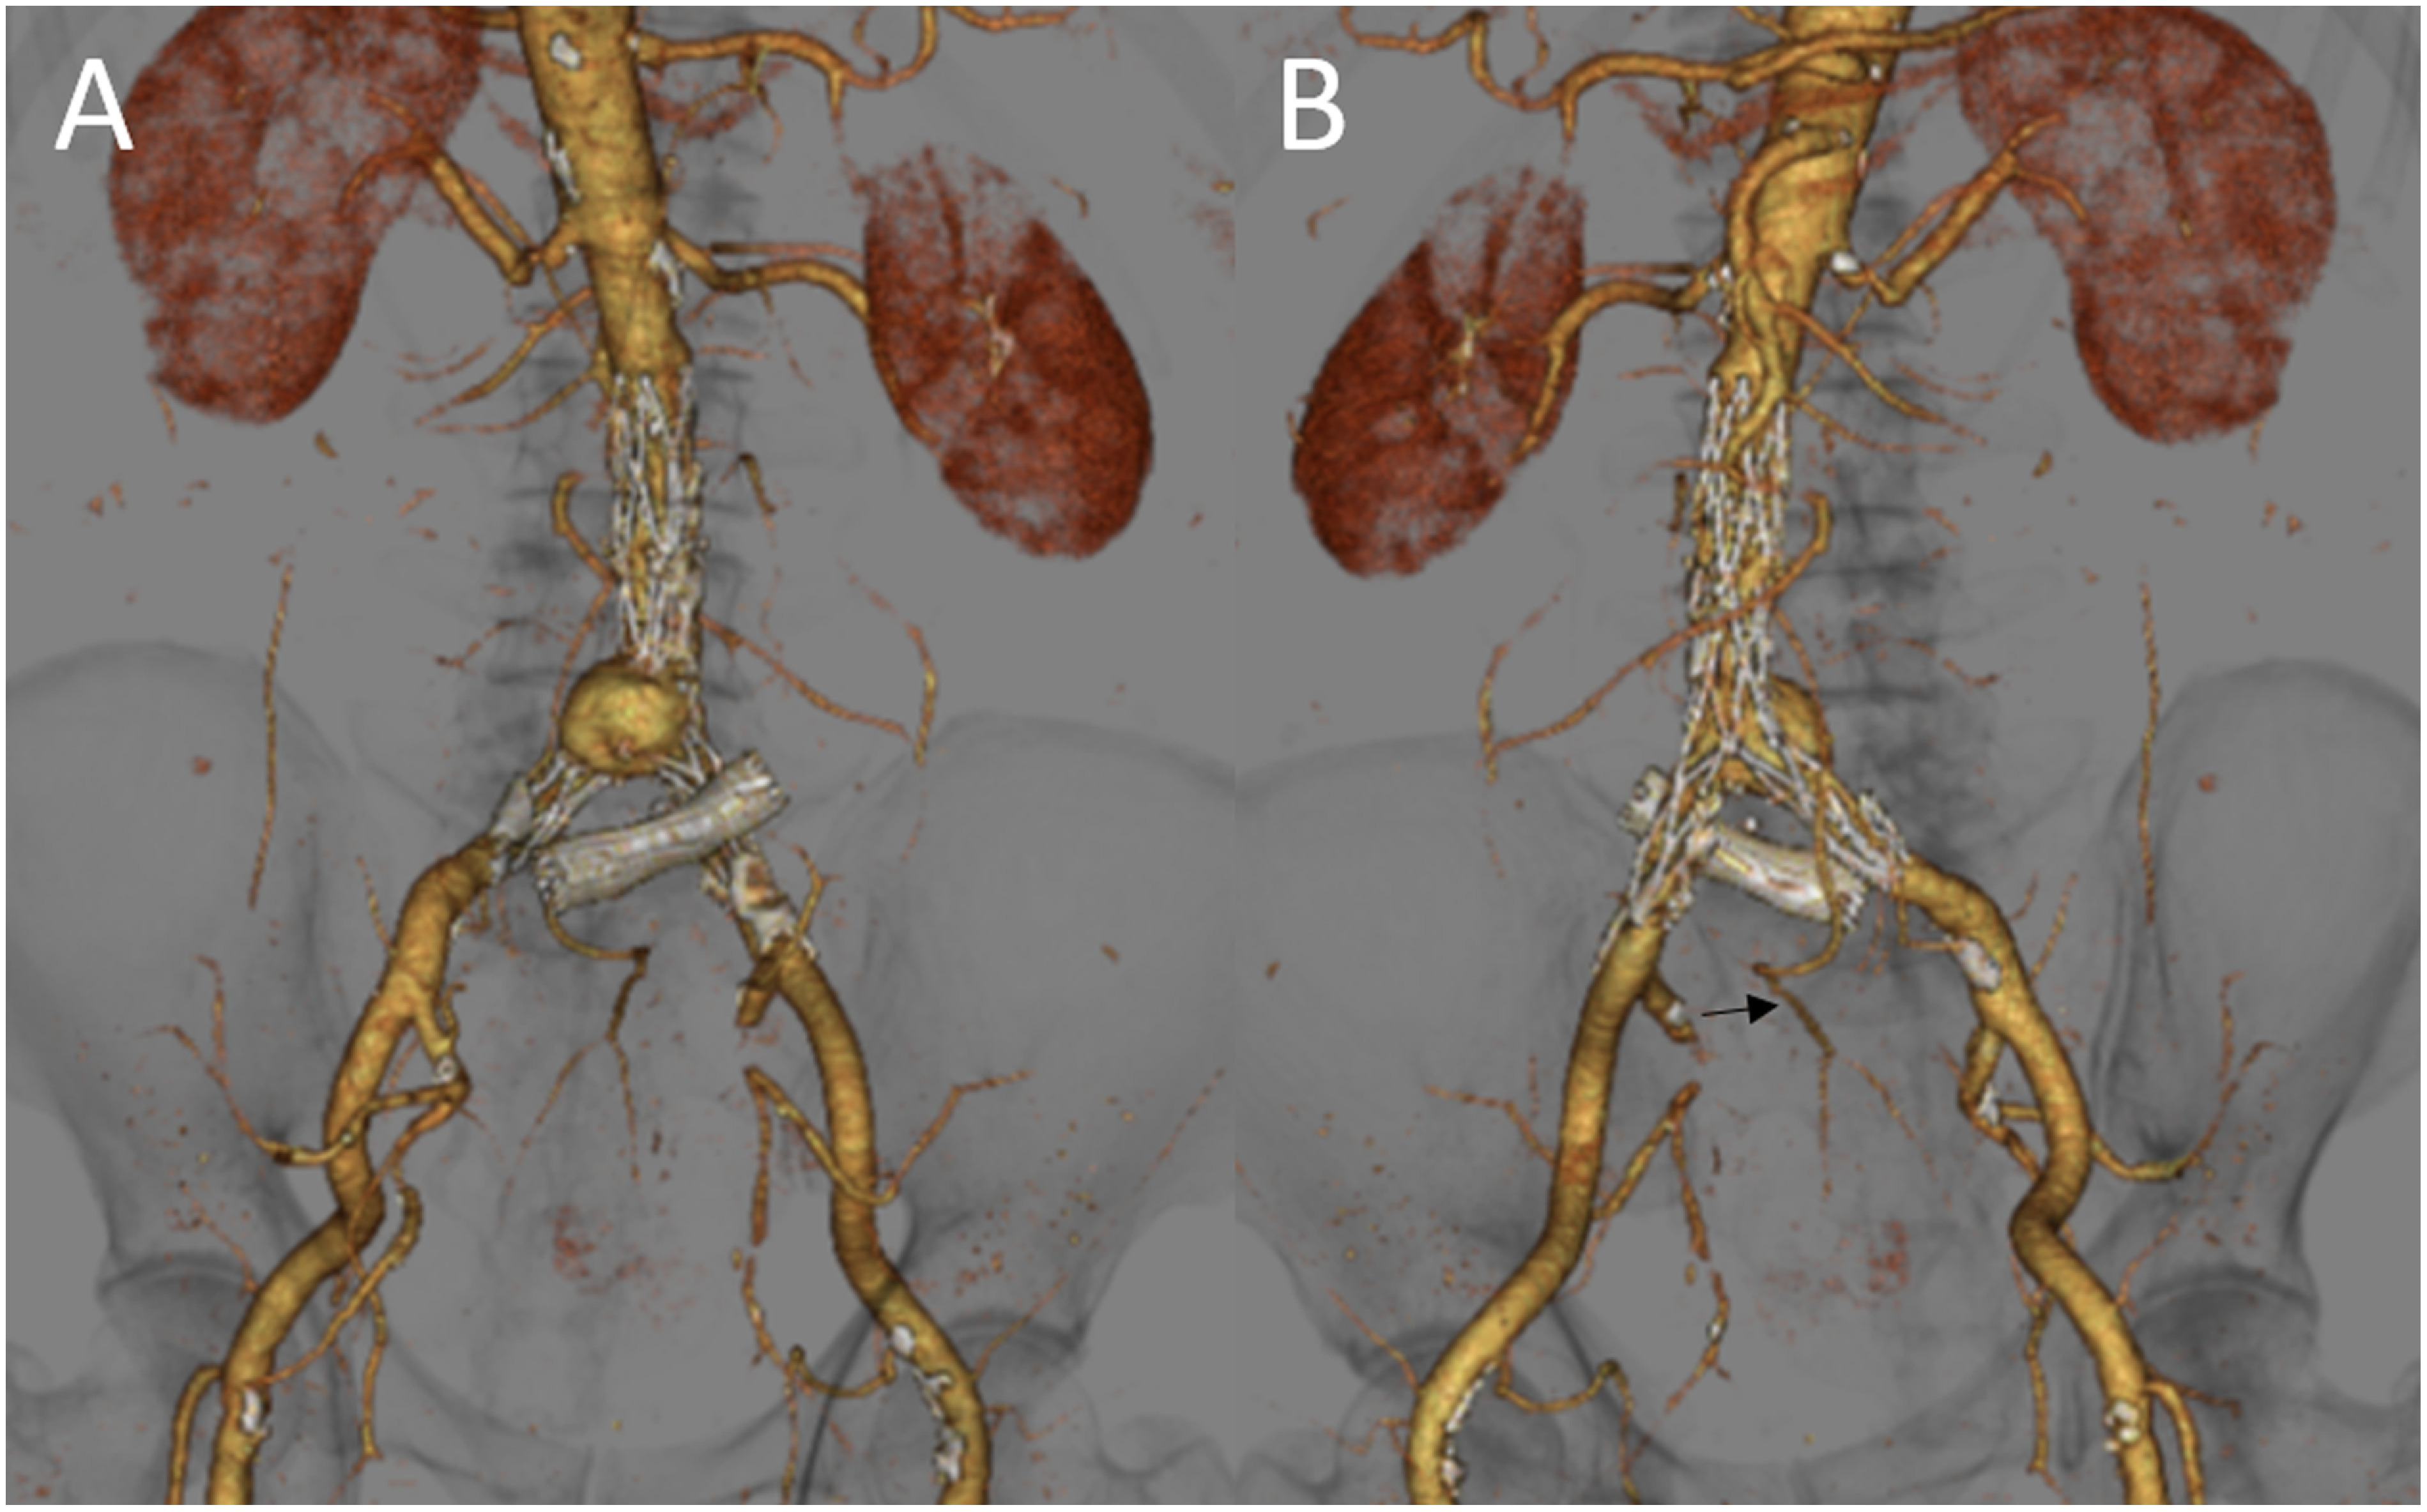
Fig. 3 Postoperative three-dimensional computed tomography (CT) findings. (A) Findings from the ventral aspect. The aneurysm and left iliac vein are covered with stent grafts without any fistula. (B) Findings from the dorsal aspect. The median sacral artery is fed by the inferior mesenteric artery, as indicated by the arrow.
